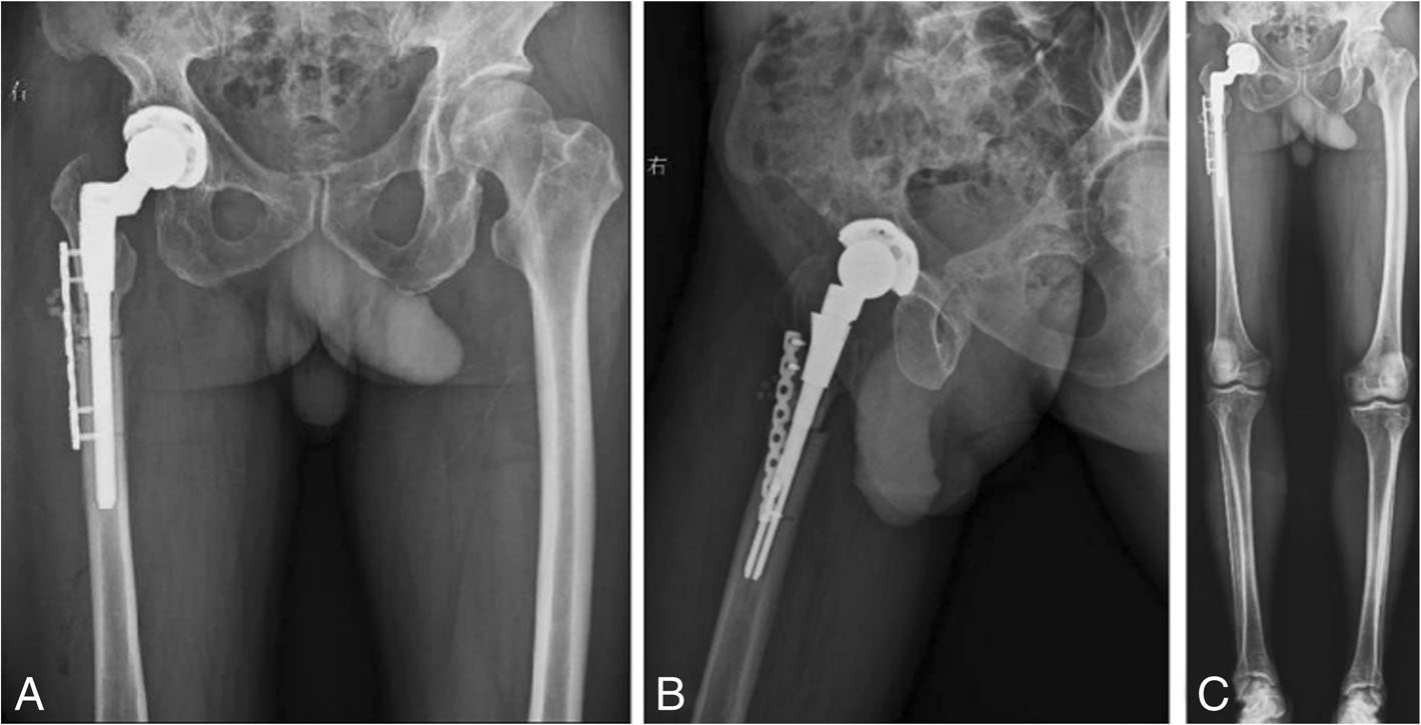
Radiographs of the right hip and both lower extremities after internal fixation was applied. (a) anteroposterior view of the right hip, (b) lateral view of the right hip, (c) anteroposterior view of both lower extremities
